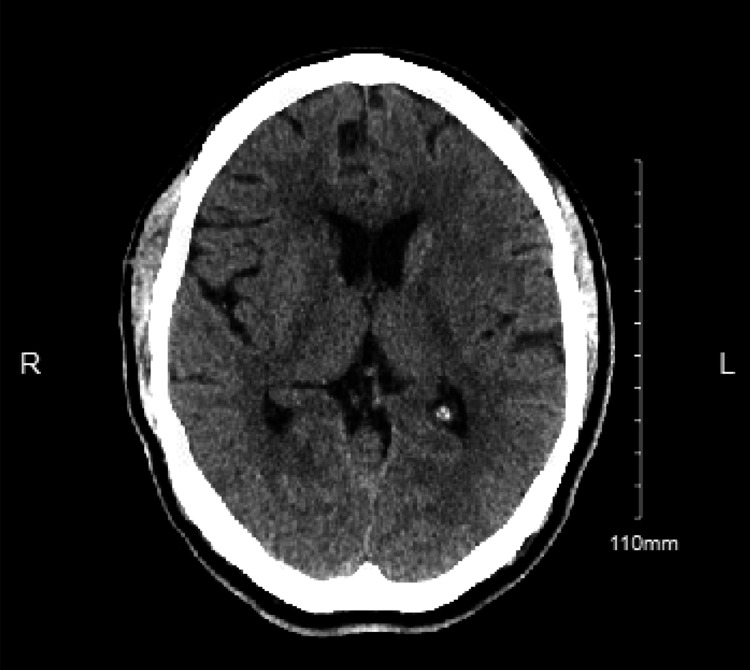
Day 0 CT of head w/o contrast. Abnormal FLAIR signal in the right caudate and left basal ganglia, with corresponding diffusion restriction in the right caudate and bilateral corona radiata/centrum semiovale white matter. Some T2 shine through is noted involving the right caudate. FLAIR: Fluid-attenuated inversion recovery
